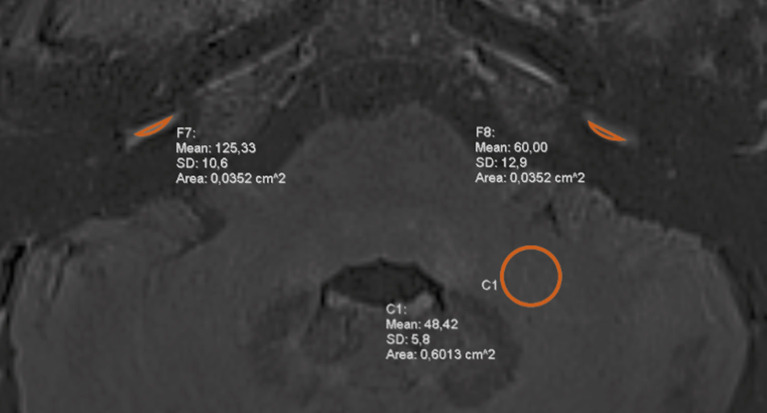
Signal intensity measurements at 4 h-delayed contrast-enhanced 3D FLAIR MRI. Symmetrical regions of interest (ROI) were drawn in the basal cochlear turn of each ear and a circular ROI was placed in the left middle cerebellar peduncle. The measurements were used to calculate the signal intensity ratio (SIR).
